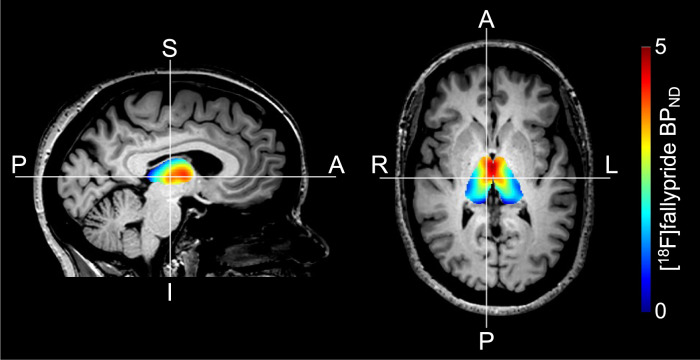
Thalamic [^18^F]fallypride BP_ND_. Representative example of [^18^F]fallypride BP_ND_ values in the thalamus overlaid on a T1-weighted image.
